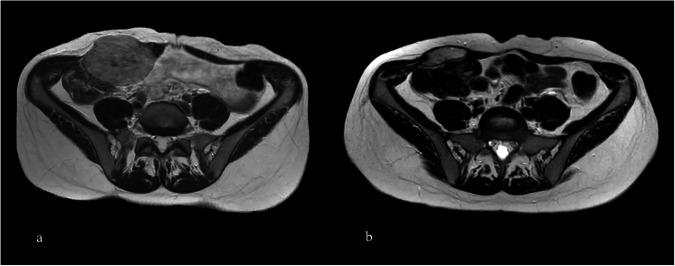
Response assessment with M-RECIST in patients with non-contrast MRI (complete response). For patients without adequate contrast-enhanced acquisitions at the latest available follow-up, tissue responses were assigned after a combined evaluation of T1, T2 and DWI changes after treatment, together with a retrospective evaluation of contrast-enhanced T1-weighted acquisitions from available priors. Follow-up imaging in this patient demonstrated extensive intralesional colliquation and a hypointense fibrotic capsule. The case was classified as CR according to M-RECIST and SD according to RECIST 1.1. a Baseline T2-weighted images; b T2-weighted images after treatment
